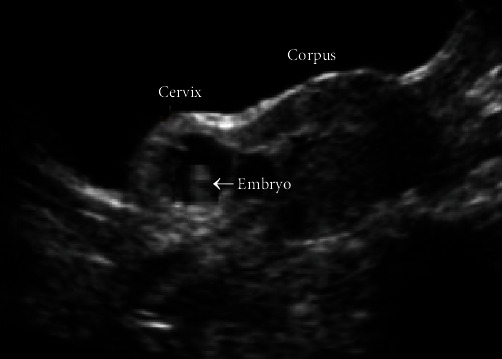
Ultrasound findings of scar pregnancy.
